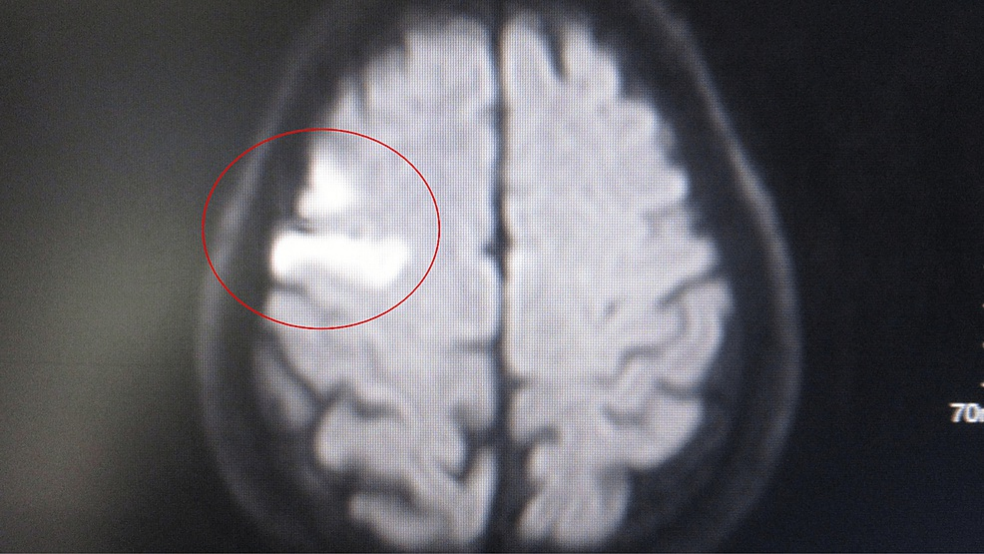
A 1.5 Tesla magnetic resonance imaging of the brain was performed without intravenous contrast. The magnetic resonance imaging (1.5 Tesla) showed an area consistent with acute infarct that appears white (within a red oval). An area of restricted diffusion in the right middle cerebral artery territory with corresponding abnormality and apparent diffusion coefficient map consistent with acute infarct. This is small in size involving the lateral right frontal cortex. The transverse relaxation time (T2) and fluid-attenuated inversion recovery (FLAIR) hyperintensity within the area of infarct was consistent with an infarct of greater than 12 to 24 hours in age. There was no evidence of hemorrhagic transformation.
